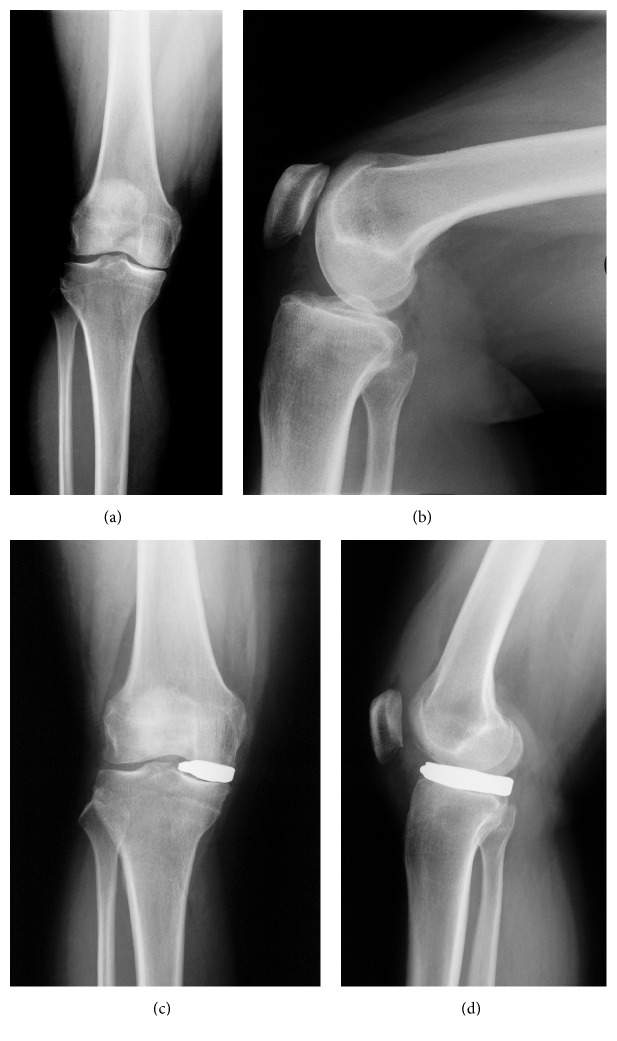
Patient-specific interpositional knee device (ConforMIS iForma), pre- and postoperative AP and lateral radiographs.
